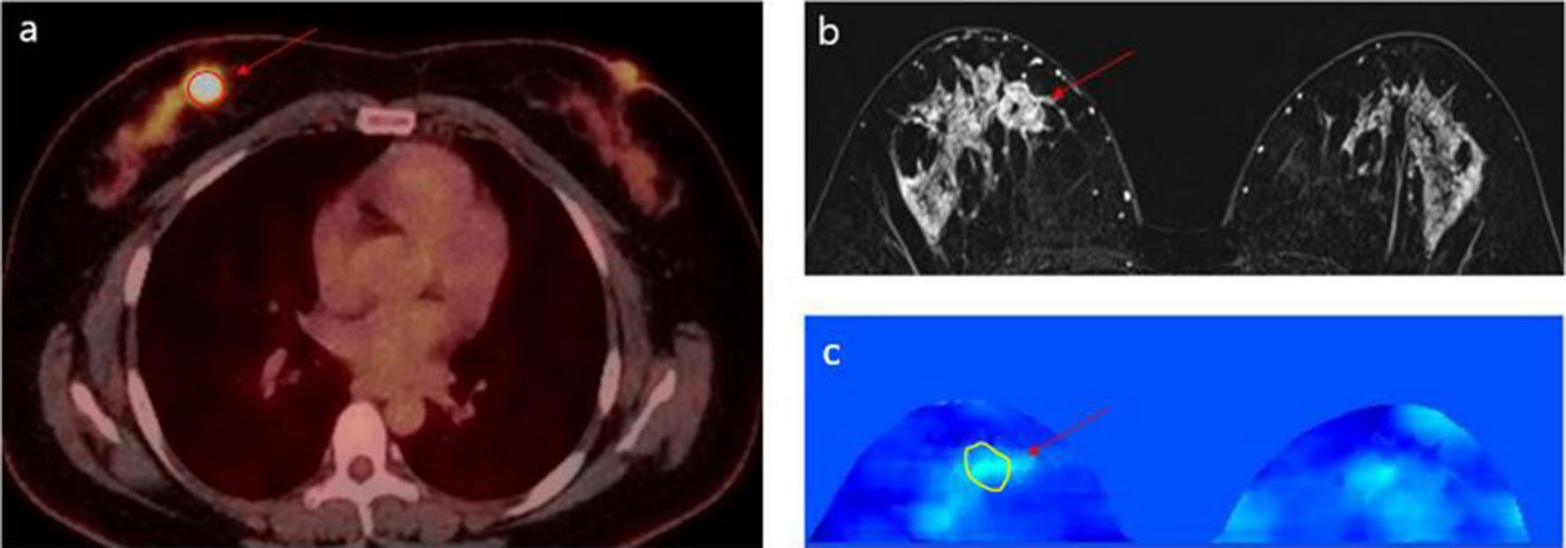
Images of a 41-year-old woman with a 2.2 cm luminal-type invasive ductal carcinoma without lymphovascular invasion in right breast. (a) ^18^F-FDG PET/CT shows a high tumor SUVpeak value of 9.13 (arrow). (b) T1-weighted contrast-enhanced subtraction image shows an irregular heterogeneously enhancing mass (arrow) in the marked background parenchymal enhancement. (c) Conductivity map shows a relatively low mean conductivity value of 0.06 S/m (arrow). While SUV showed high value despite the lack of lymphovascular invasion, mean conductivity showed relatively low value.
